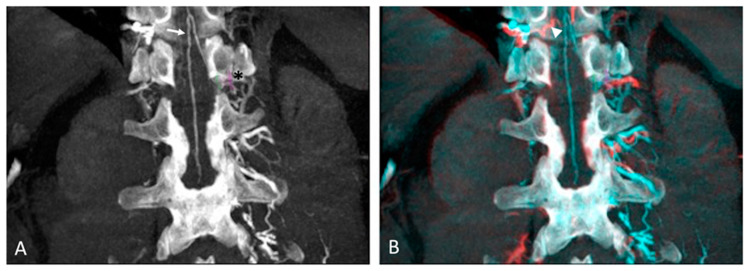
Anterior spinal and Adamkiewicz arteries detection on intra-aortic CBCT coronal images with maximum intensity projection: (A) Arterial phase of CBCT showing the anterior spinal artery with its characteristic “hairpin” curved vessel (arrow), supplied by 12th left intercostal artery (Asterix). Note the purple circle placed as a landmark on the intercostal foramina. (B) Registered arterial and delayed phases of CBCT with identification of great anterior.
